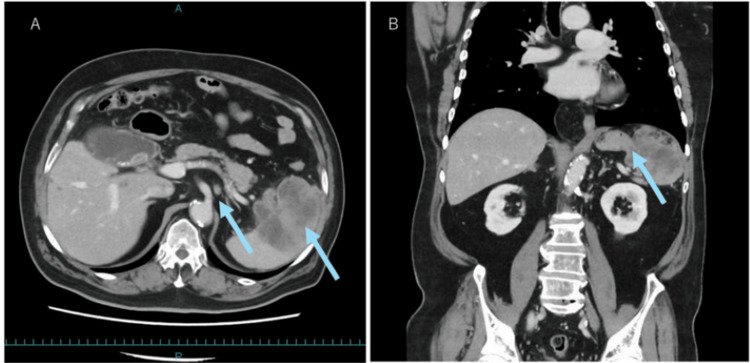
Contrast-enhanced abdominal computed tomography images (A) The coronal view shows a large, heterogeneously enhanced mass in the spleen (arrows). (B) The axial view demonstrates the 9 × 8 cm splenic tumor with suspected gastric wall invasion and a swollen lymph node adjacent to the celiac artery (No. 11p) (arrow).
